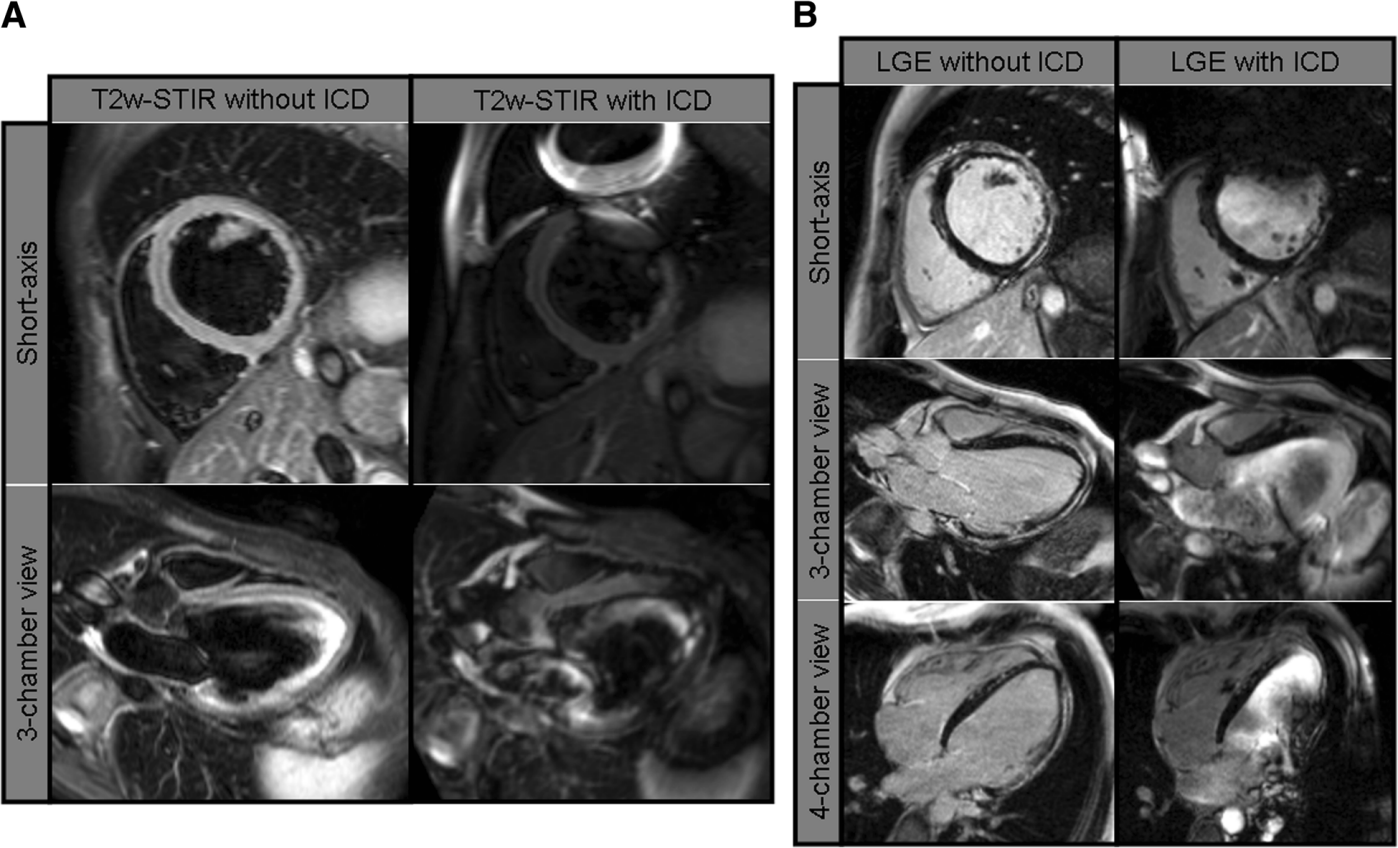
Edema and contrast images prior to and post ICD-implantation. A. T2-weighted Edema and contrast images prior to and post ICD-implantation. T2-weighted “edema” images (T2w-STIR spin-echo) in midventricular short-axis and 3-chamber view before (left panel) and after ICD implantation (right panel). Similarly to the cine-CMR, artifacts from the ICD render the anterior and antero-lateral segments not assessable (right panel). B. Late gadolinium enhancement (LGE) images prior to and post ICD-implantation. Late gadolinium enhancement (LGE) images in mid-ventricular short-axis, 3-chamber and 4-chamber views before (left panel) and after ICD implantation (right panel).
